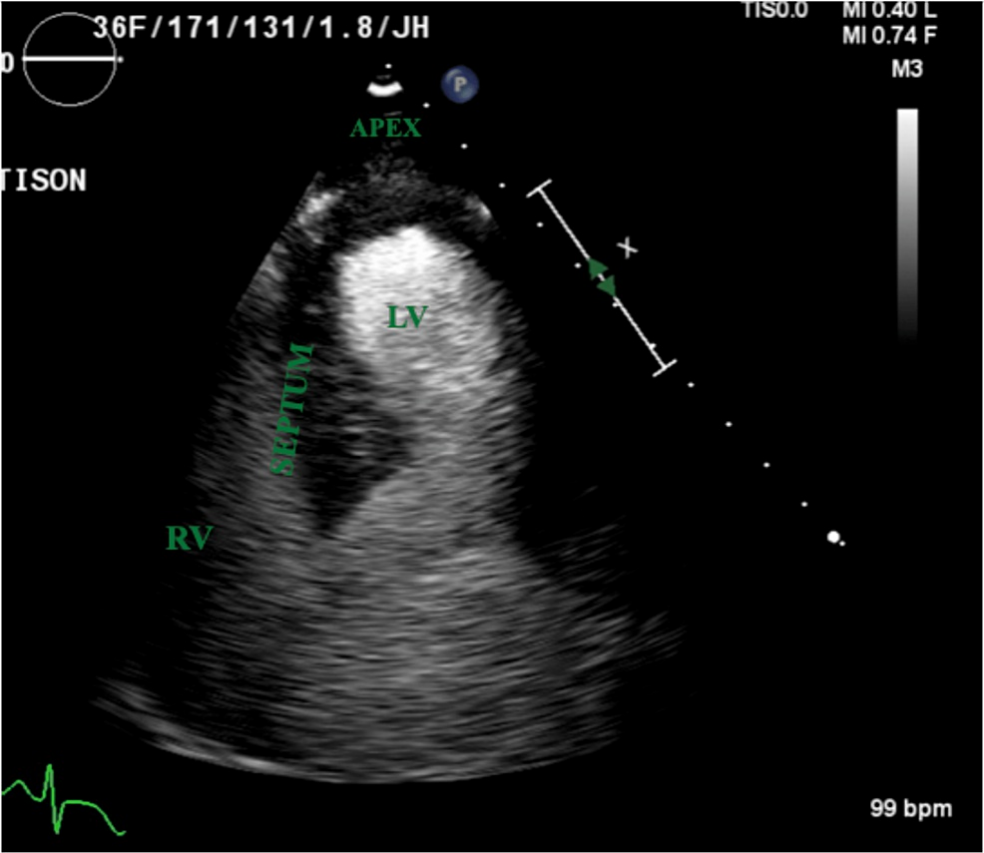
Echocardiogram showing apical ballooning in systole LV: left ventricle, RV: right ventricle
